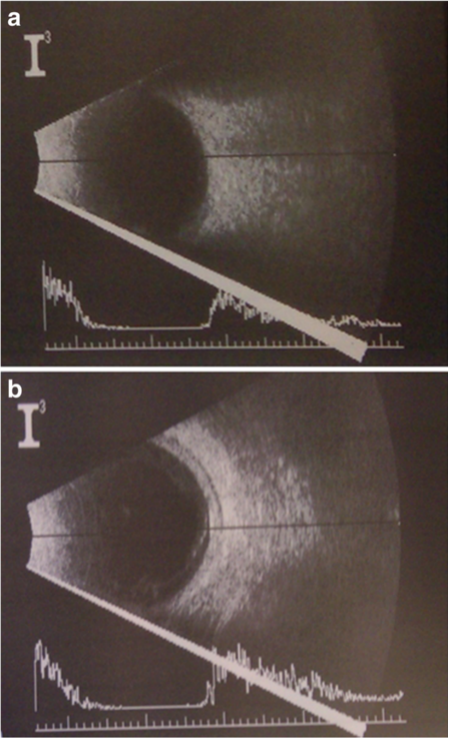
Ultrasonography (B-scan), left eye, day 3. a First look at 8.00 am; vitreous with no evidence of infiltration, no retinal detachment. b Second look at 2.00 pm; vitreous appears condensed and infiltrated at the posterior hyaloid membrane compared to the first look of the day, no retinal detachment
